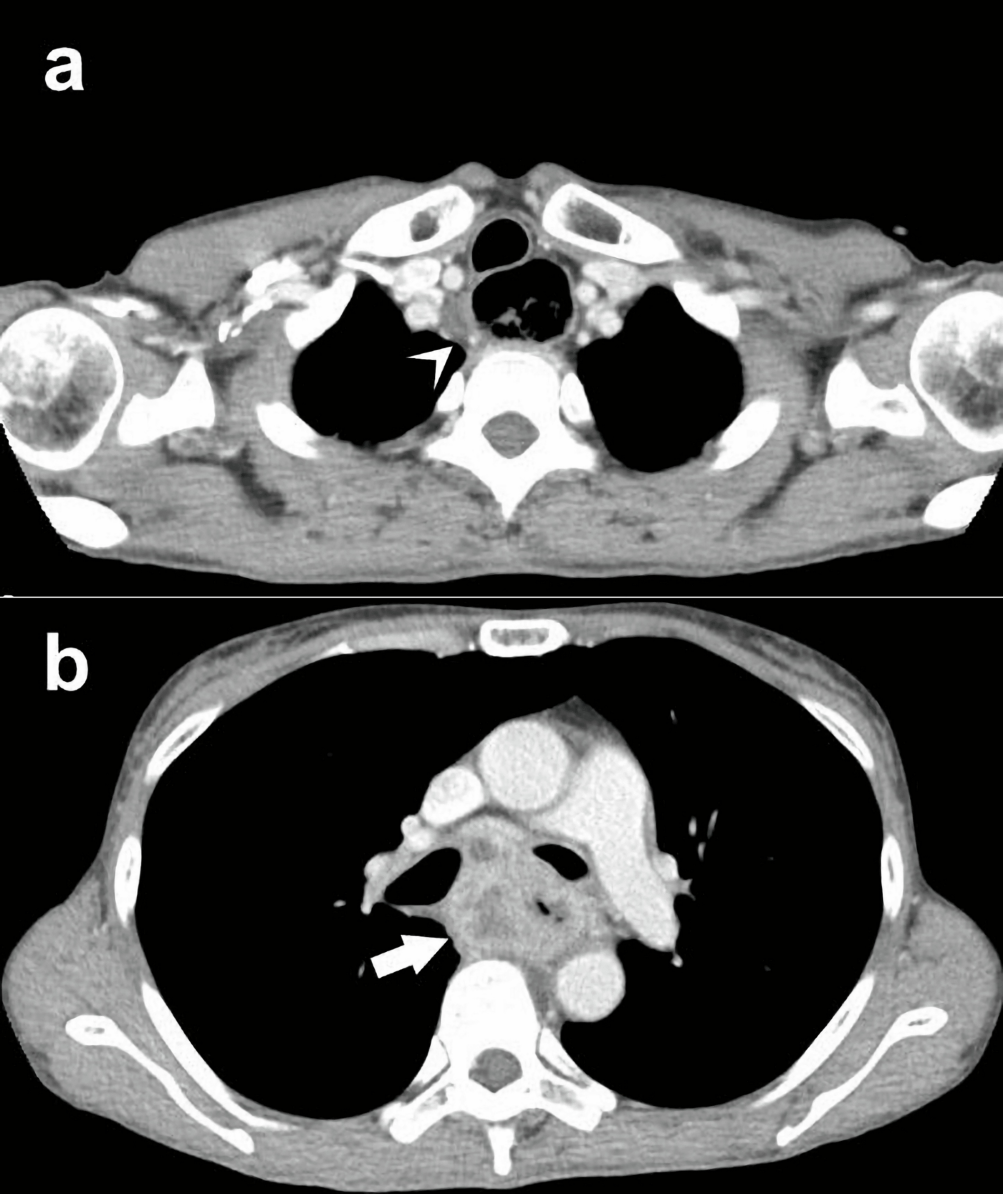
CT findings before treatment (a) Recurrent nerve node metastasis (arrowhead) and esophageal dilatation. (b) 4 cm esophageal mass with suspected bronchial invasion (arrow). Staging was determined according to the TNM Classification of Malignant Tumours, 8th Edition [2]. CT: computed tomography
